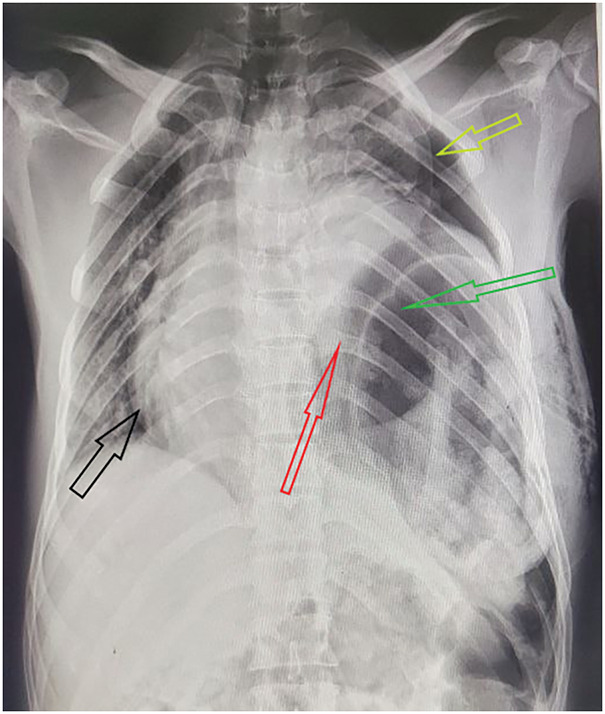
Anteroposterior CXR demonstrating left pneumothorax with collapse of the left lung (yellow arrow), severe rightward mediastinal shift (black arrow), loss of the left diaphragmatic contour, intrathoracic gastric bubble and bowel loops in the left hemithorax (red and green arrows), and the pre-existing left chest tube *in situ*. No rib fracture is visible. CXR, chest radiograph
